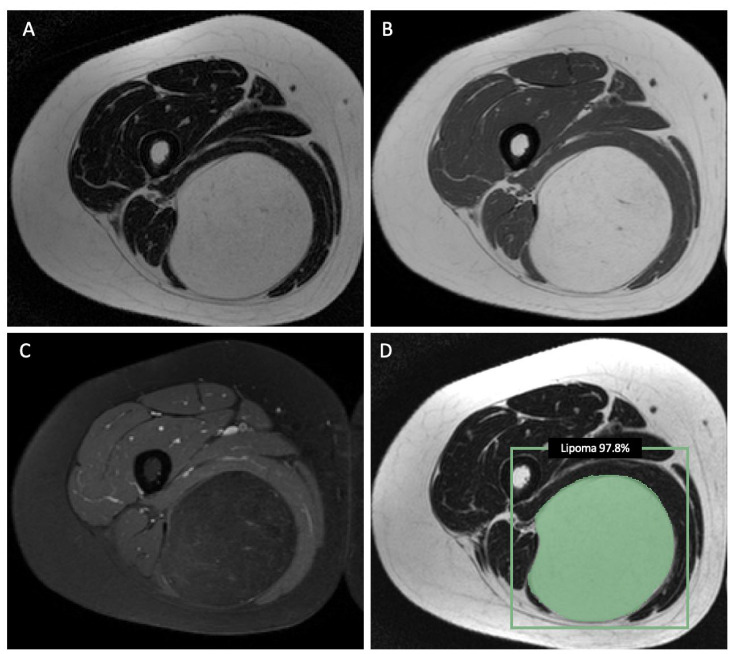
Axial T2-weighted (A) and T1-weighted (B) MR images showing a well-defined intramuscular lipomatous tumor (lipoma) in the right posterior thigh without significant contrast enhancement on the axial T1-weighted image with fat saturation (C). (D) The machine-learning model classified this tumor as a lipoma (probability of 97.8%). This was in accordance with the diagnosis made by the radiology residents and the attending radiologist.
